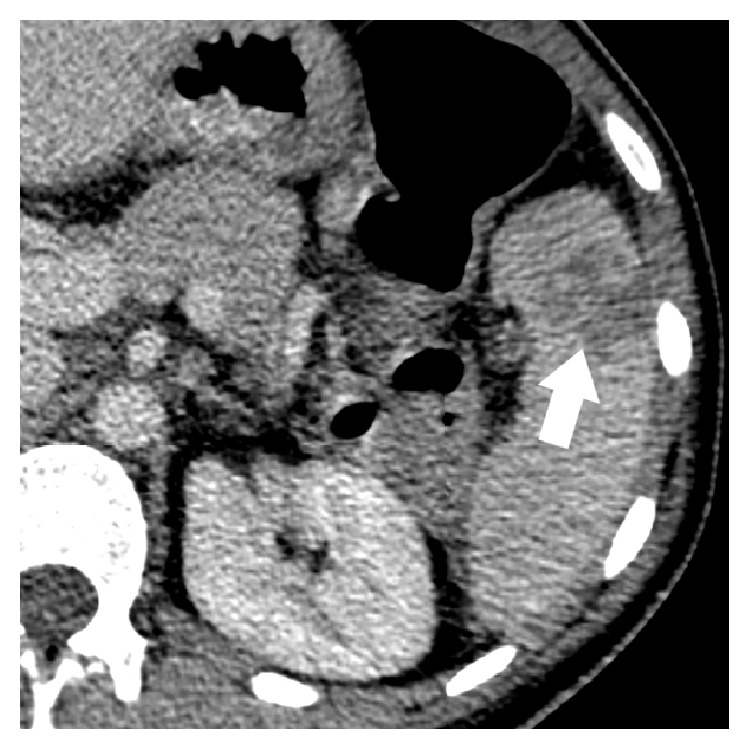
Axial contrast enhanced CT image demonstrating a heterogeneous splenic abscess (thick arrow).
